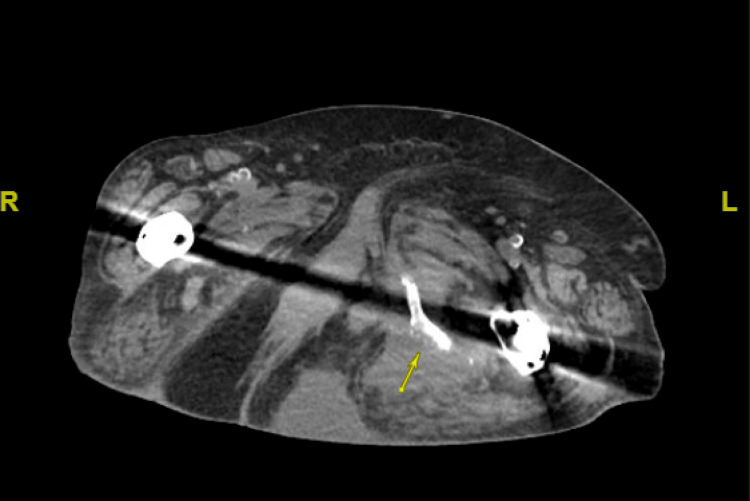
Axial non-contrast CT of the abdomen and pelvis Series 2, images #124–134, demonstrating a large complex fluid collection within the left gluteal and posterior thigh musculature (yellow arrow), measuring approximately 10 x 9 x 5.9 cm, with internal punctate calcifications. The collection extends inferiorly beyond the field of view and involves the left abductor muscles.
